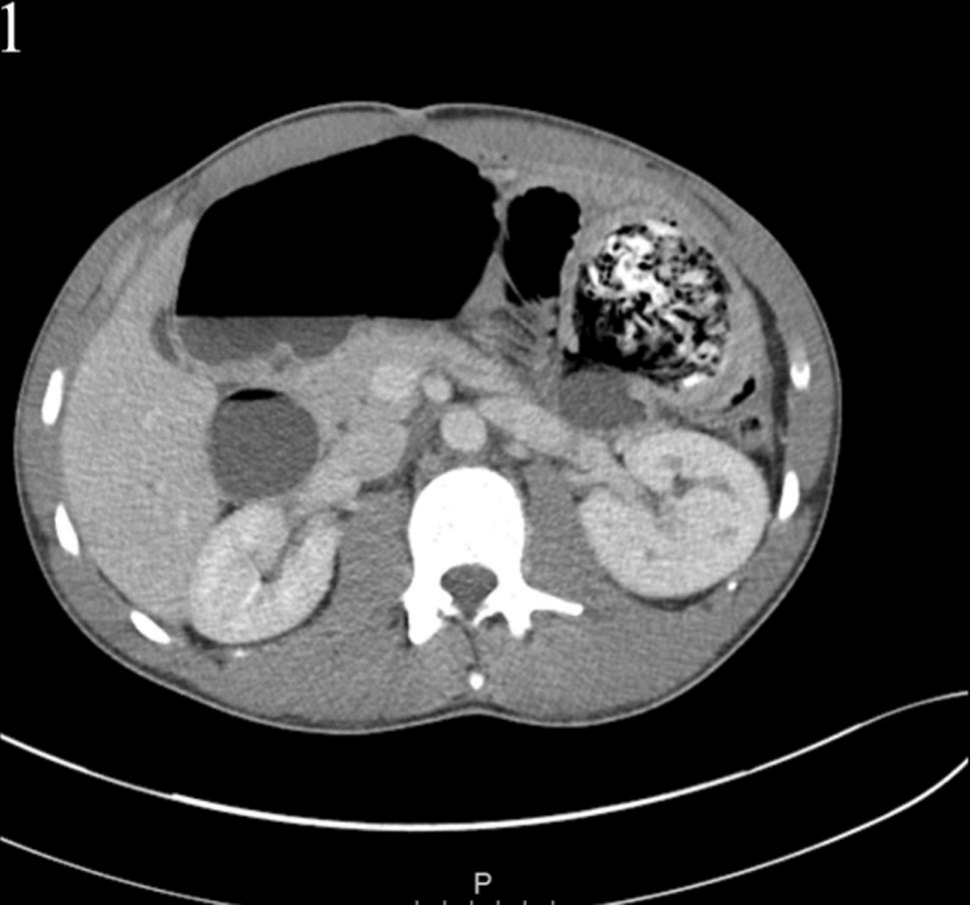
Axial CT image showing proximal duodenal and gastric distension secondary to heterogenous intraluminal mass at the duodenal jejunal flexure/proximal jejunum which is suspicious for gossypiboma
